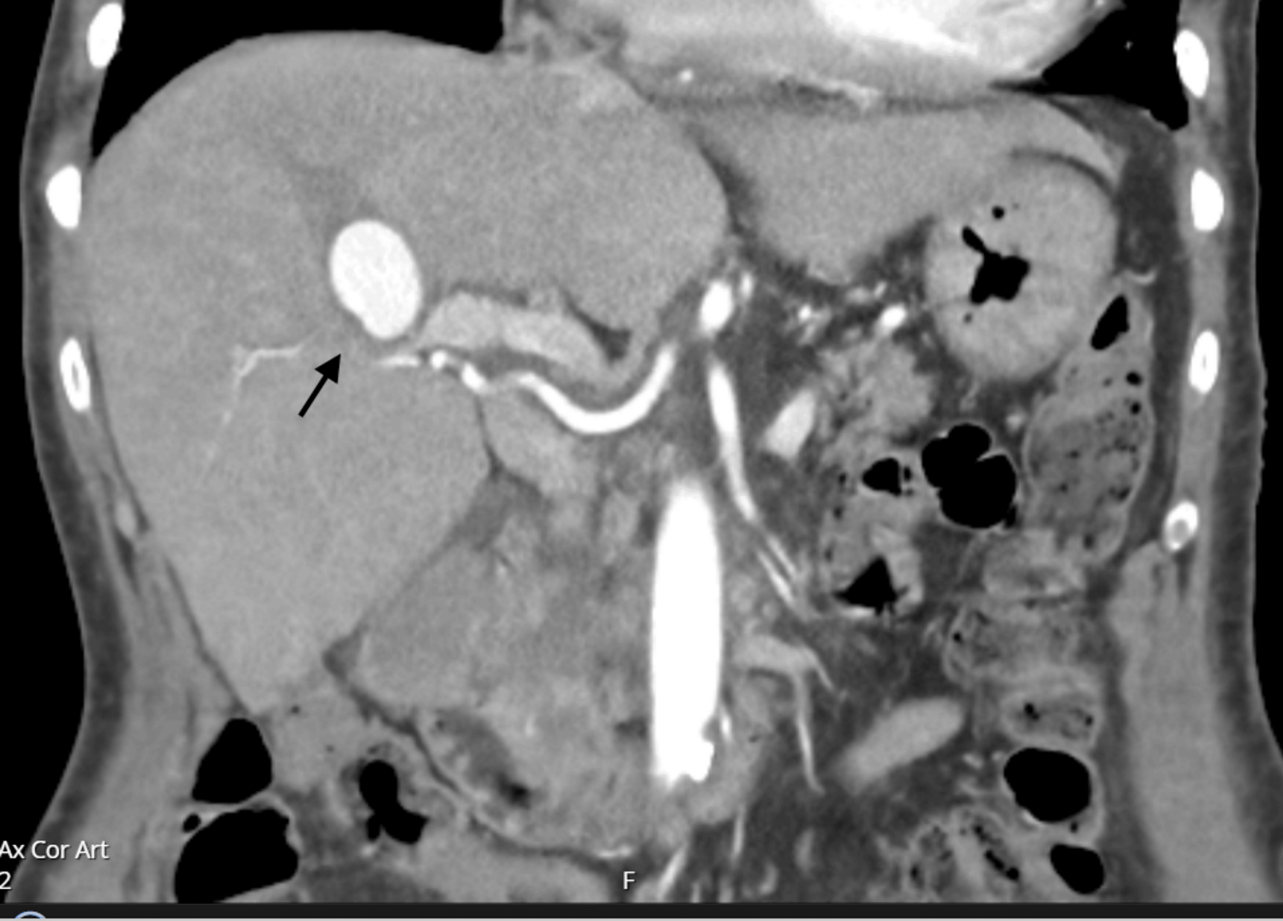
Triple phasic Computed Tomography of the liver in the coronal view identifying a 3 cm x 2.5 cm aneurysm (black arrow) arising from a branch of the left hepatic artery.
